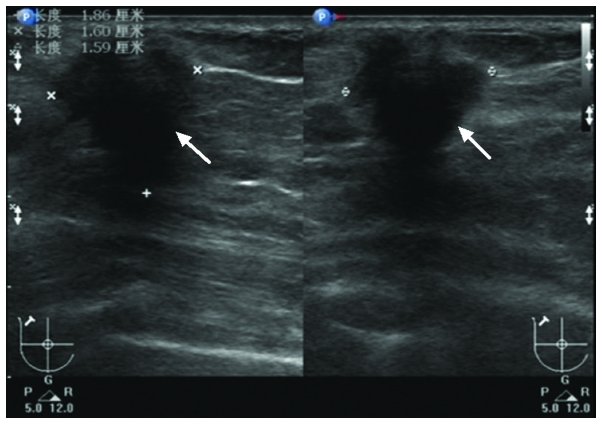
Ultrasound of the lesion showing a definite hypoechoic mass with angular margins, spiculations and an acoustic shadow posterior to the mass.
